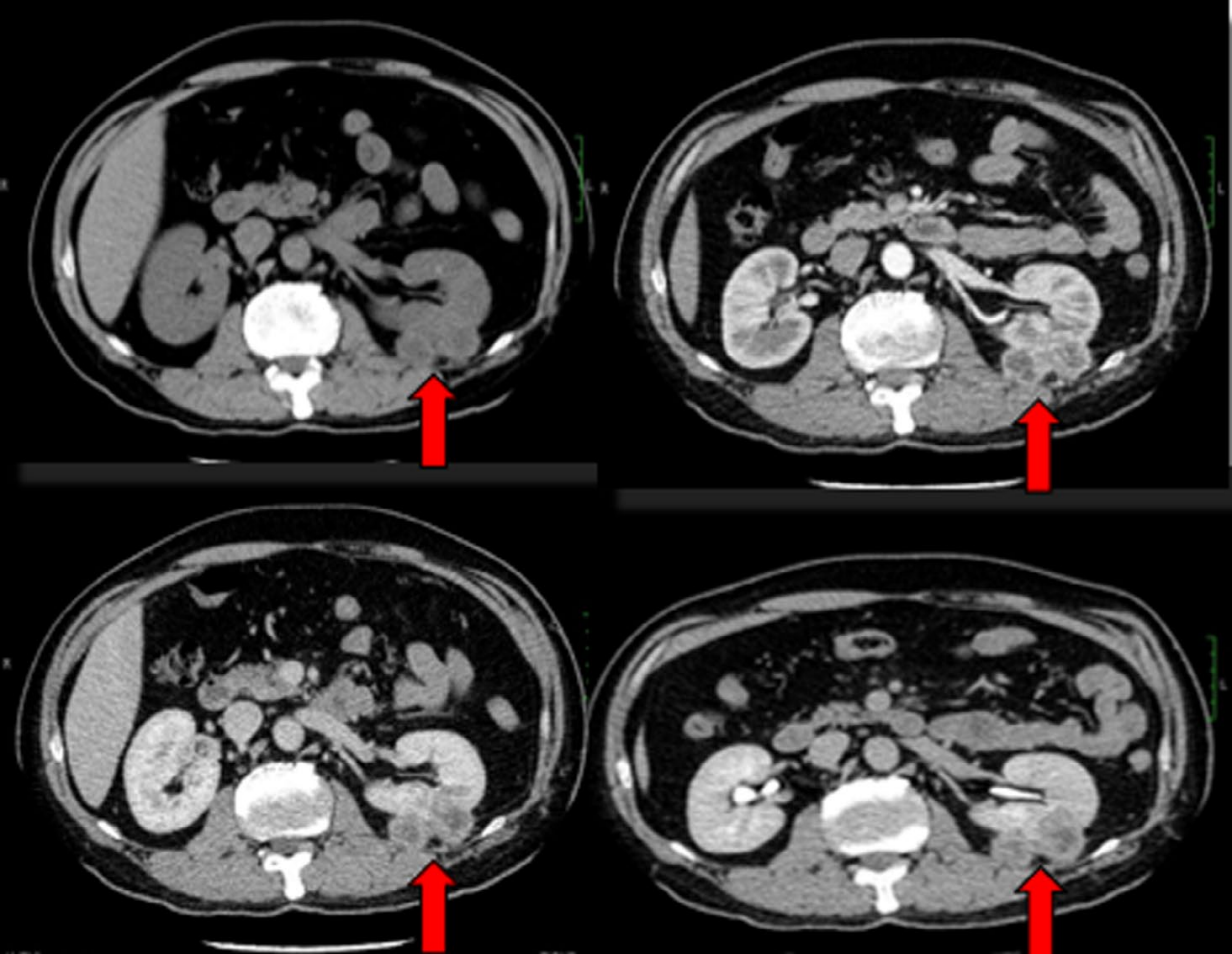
Urinary CT examination on second admission.
